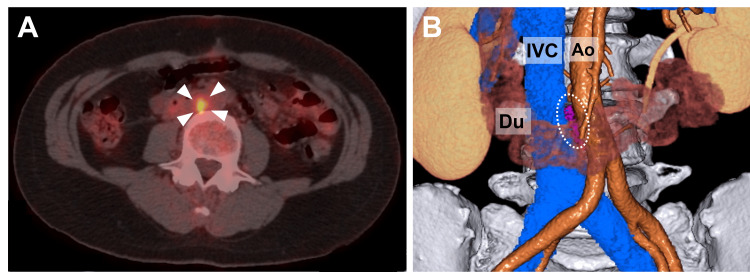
Positron emission tomography (PET) and 3D-computed tomography (CT) showing the local recurrence (A) PET revealed a small mass (1.3 × 0.8 cm) with a maximum standardized uptake value of 6.7 (arrowheads). (B) On 3D-CT reconstructed imaging, the tumor was located in the para-aortic region near the previous surgical site (dotted line). Ao: aorta; Du: duodenum; IVC: inferior vena cava
